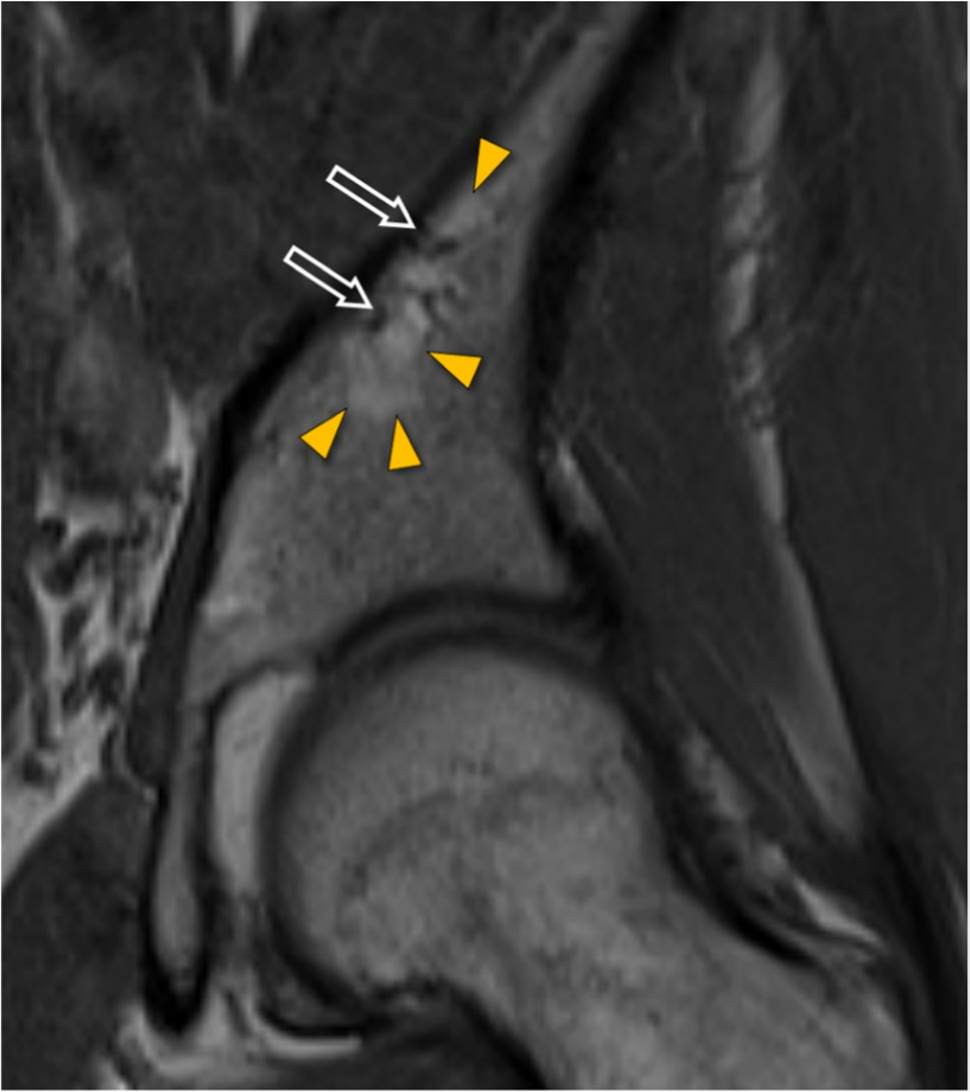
Central vessel convolute (CVC) with surrounding fat signal in a coronal T1-weighted MR image of the left pelvis in a 65-year-old female. The CVC with two main branches is indicated by outline arrows, and the adjacent area with fat signal is indicated by orange arrowheads
